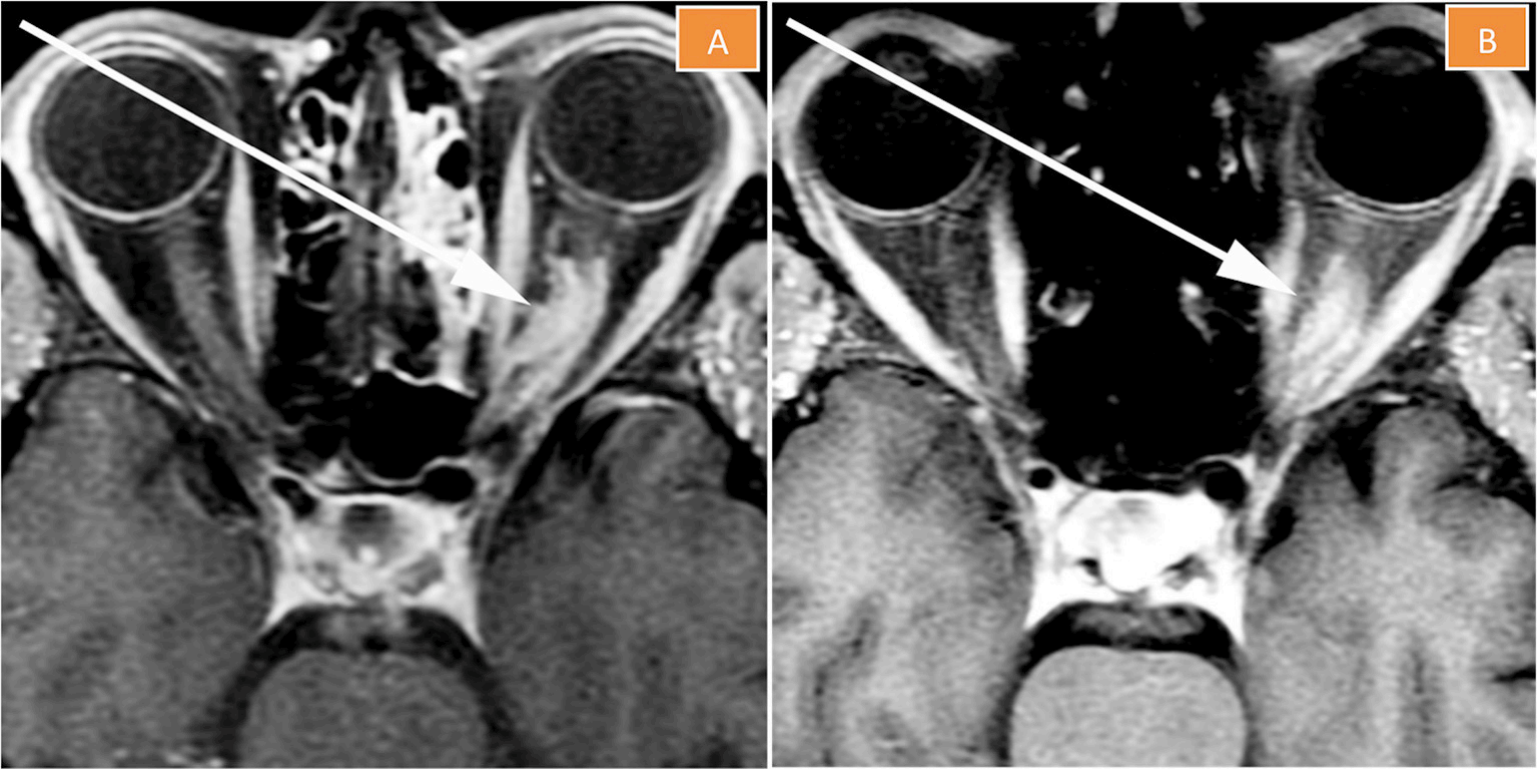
Magnetic resonance imaging of the left orbit 2 months (A) and 5 months (B) after treatment completion. The tumor is less enhancing than pre-treatment, its size is stable to slightly decreased, and “tram-tracking” is more apparent.
